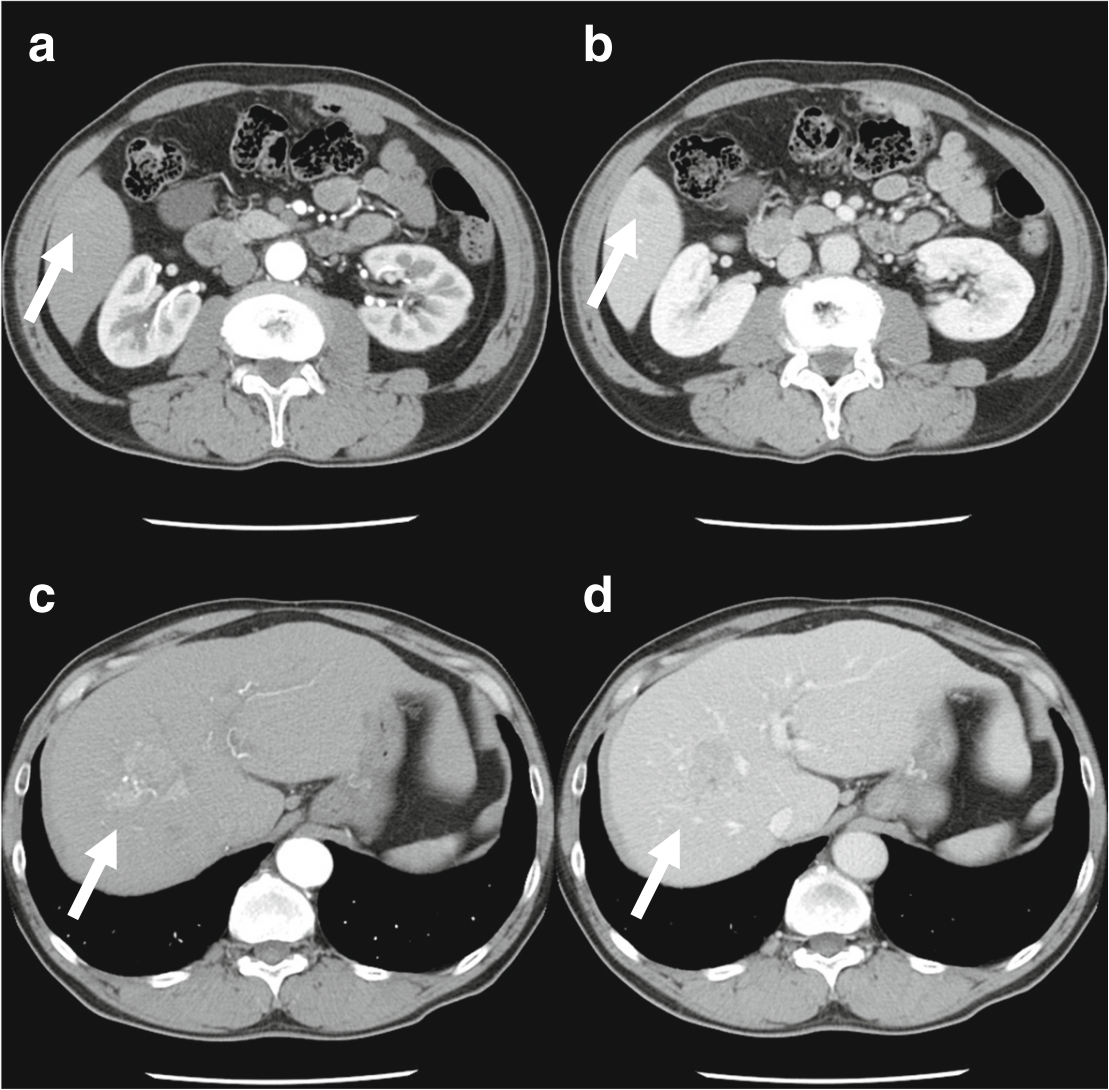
Dynamic CT findings before surgery. Dynamic CT revealed two tumors (arrows) located in a, b segment 8 and c, d segment 5. a, c Findings in the early phase. b, d Findings in the delayed phase
